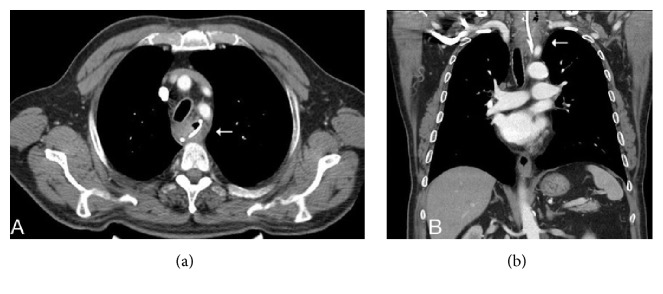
CT images obtained after placement of an endoscopic catheter, which was confirmed to be correctly located ((a) and (b), arrows).
